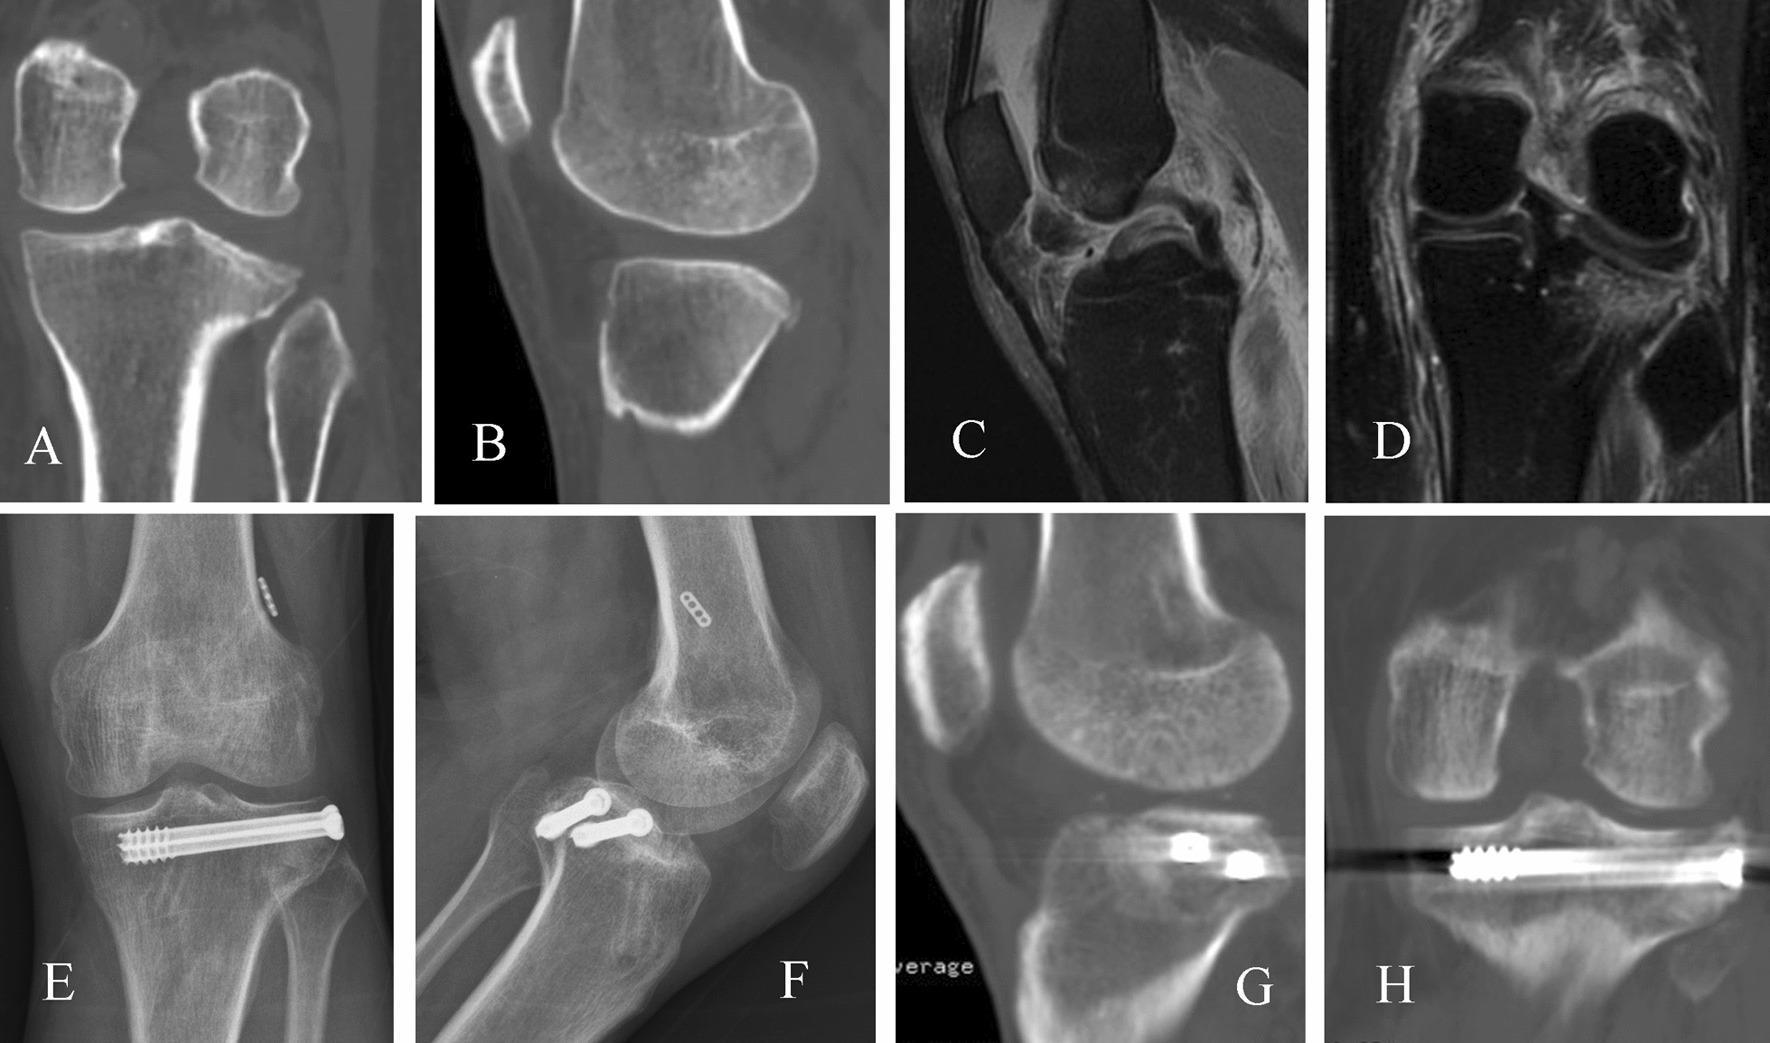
A 55-year-old male patient was admitted due to left knee pain following a fall from an electric bicycle. 3DCT scans of the left knee upon admission revealed a compressive fracture of the posterolateral tibial plateau. The coronal view demonstrated a significantly increased lateral inclination of the lateral tibial plateau (A), and the sagittal view displayed an increased posterior inclination (B). The sagittal view of the MRI revealed an acute ACL tear (C), while the coronal view of the MRI also indicated a lateral tibial plateau compression fracture (D). The patient underwent a one-stage procedure involving ACL reconstruction and arthroscopically assisted reduction and fixation of the PTPCF using two cannulated screws without bone grafting. Fourteen months post-surgery, the fracture fragments exhibited successful union with no significant reduction loss (E–H)
